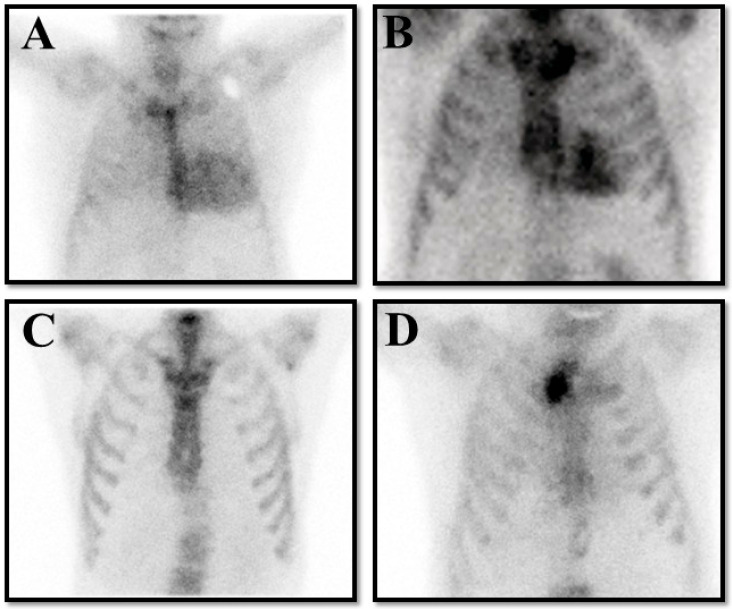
The panel represents four possible results on bone scintigraphy in patients with clinical suspicion of transthyretin cardiac amyloidosis (ATTR-CM). (A) is a true positive result compatible with Perugini grade 3. (B) is a false positive result in a patient with hypertrophic cardiomyopathy. (C) is a false negative result in a patient with hereditary ATTR-CM for a Val30Met pathological variant. (D) is a true negative result in a patient without ATTR-CM.
